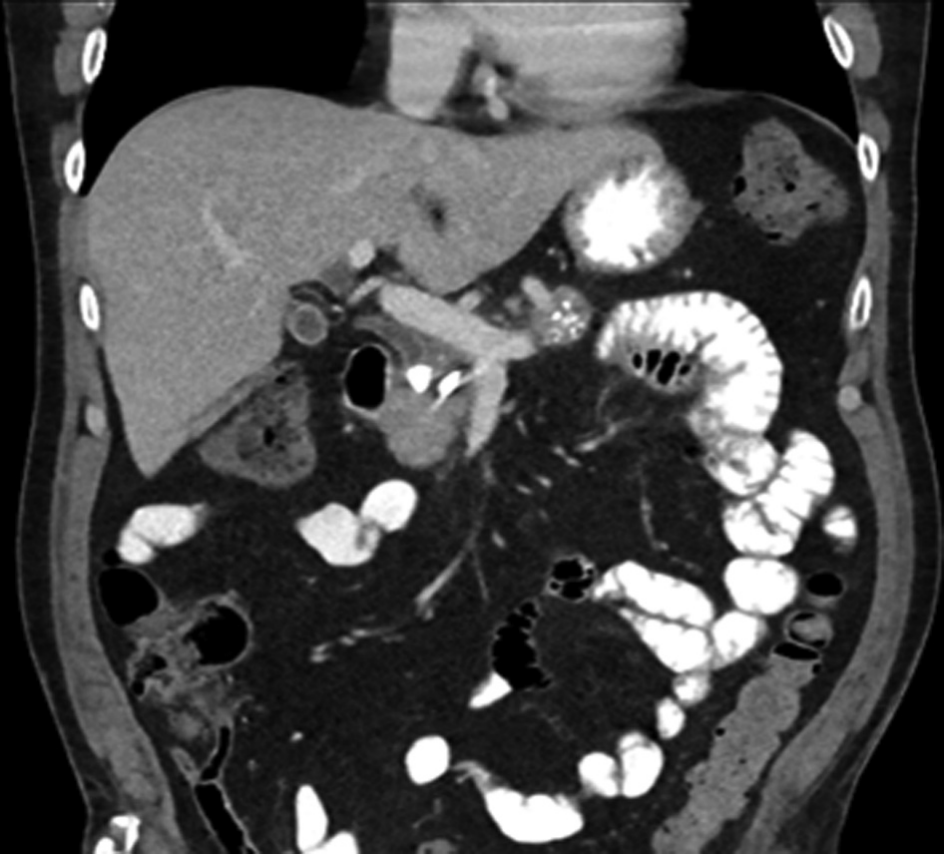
CT of the abdomen showing compression of the bile duct by the pancreatic stone.
